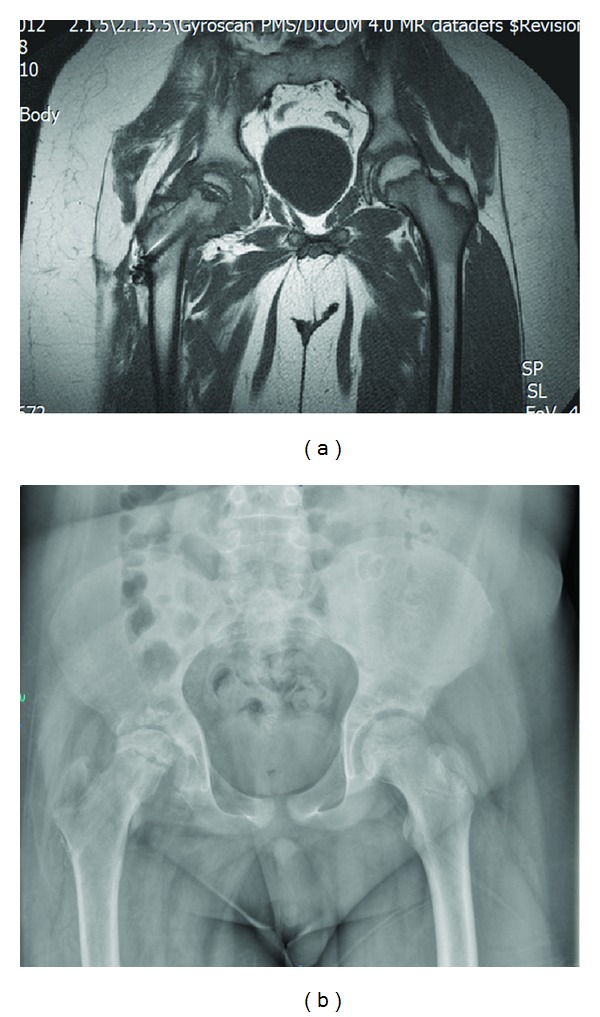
(a) One-year follow-up magnetic resonance imaging study shows no signs of avascular necrosis; (b) the screw removed after one year.
